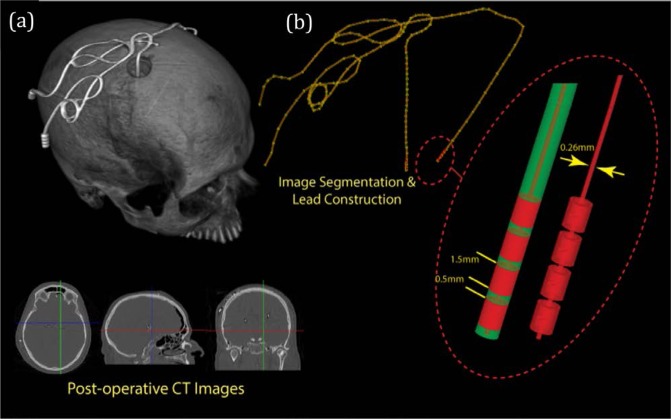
(a) Three-dimensional reconstruction of intra-operative CT images from a DBS patient, with representative axial, coronal and sagittal images shown below. (b) Segmentation of DBS lead, with zoomed-in region showing in detail how the lead tip was modelled.
